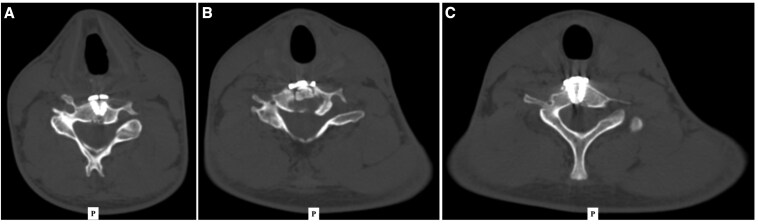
Postoperative axial CT scan images of the cervical spine at levels C5 (A), C6 (B), and C7 (C), following surgical fusion and stabilization. The patient underwent posterior cervical fusion of C5-C7 with pedicle screw fixation. All images demonstrate appropriate pedicule screw placement and preserved alignment. The letter “P” denotes the posterior direction for anatomical orientation.
